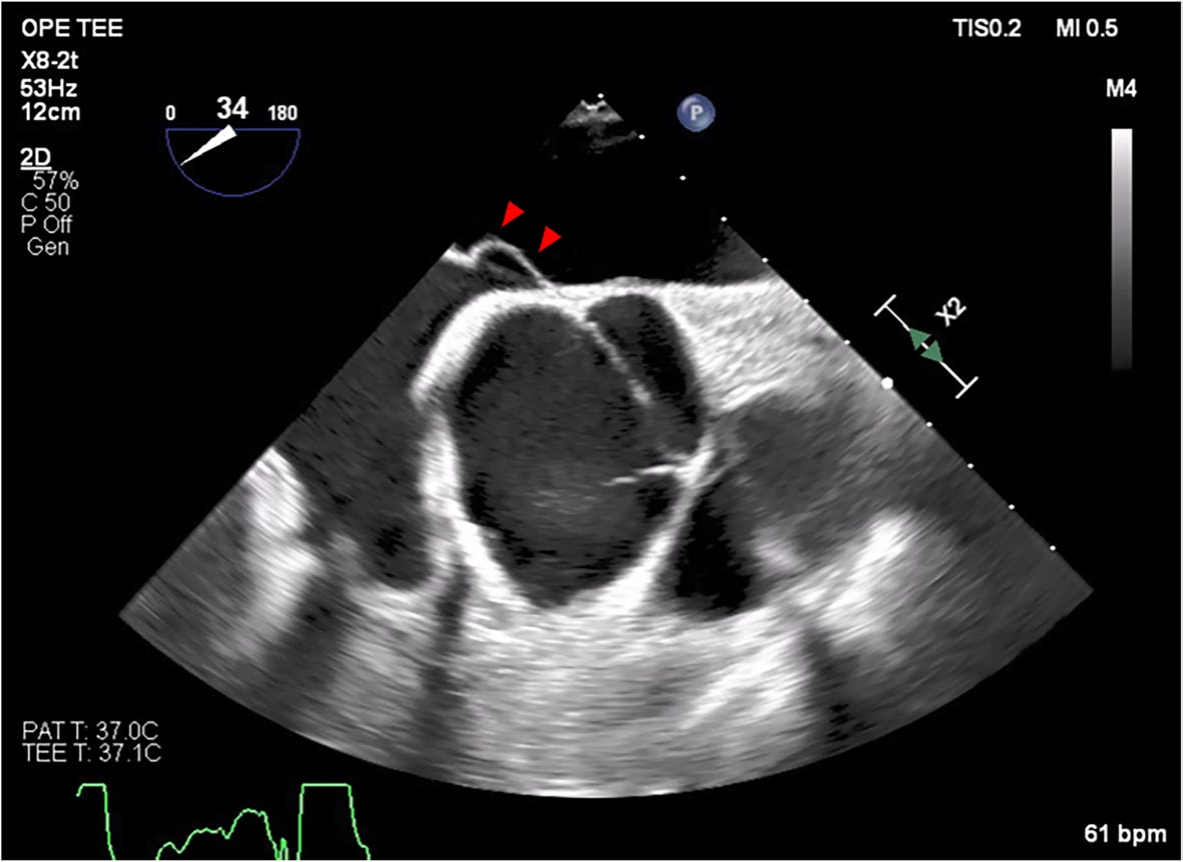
Intraoperative transesophageal echocardiography: aortic valve short axis view. An atrial septal aneurysm with a 10-mm deviation of the atrial septal tissue into the left atrium (arrowhead)
